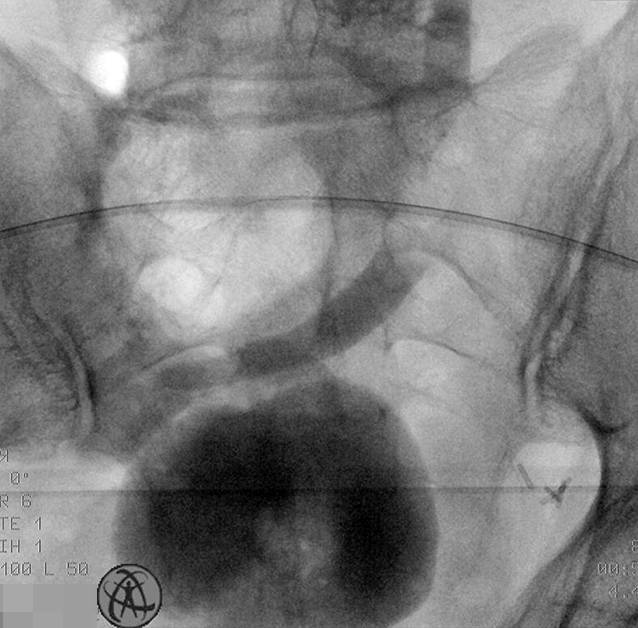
Der Patient findet sich derzeit in regelmäßiger Nachsorge mit sonographischen Verlaufskontrollen, Blutgasanalysen und Laborkontrollen.
